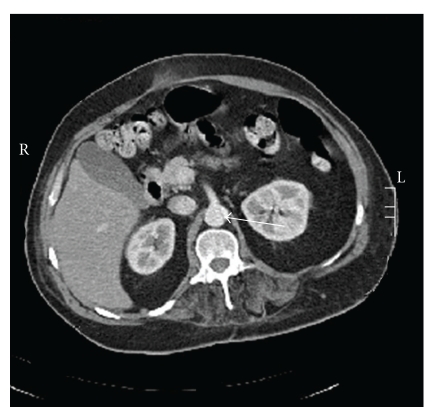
Post-op contrast CT abdomen showing supraceliac aortic dissection (white arrow).
